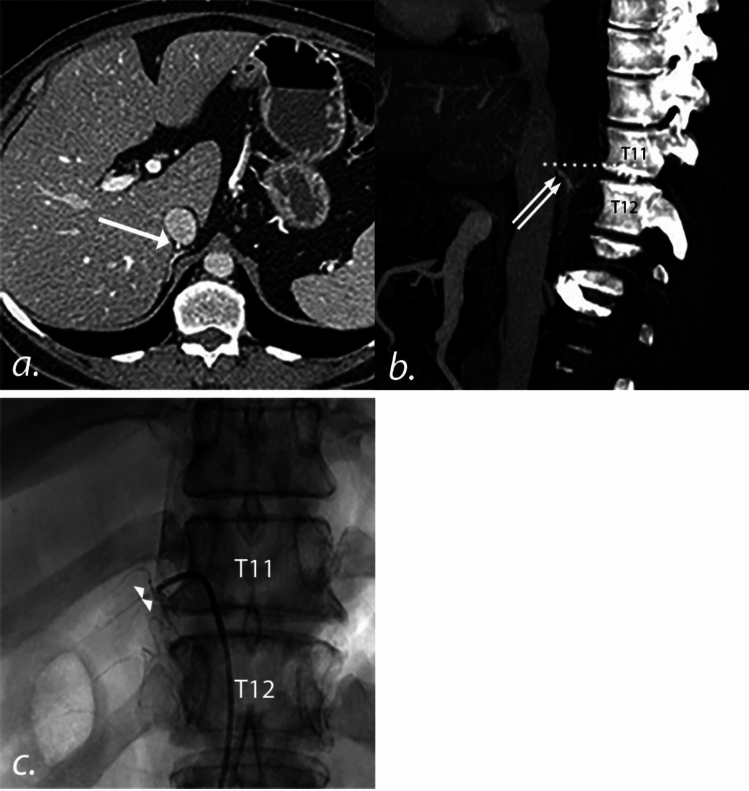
CT imaging and intraprocedural fluoroscopic images during AVS. a Axial CT images from a pre-AVS CT scan demonstrates the origin of the RAV (*arrow*). b Sagittal reformatted CT image from the pre-AVS CT scan demonstrates that the RAV origin (*double arrow*) maps to the lower third of the T11 vertebral body (dotted line). c Intraprocedural fluoroscopic image during AVS demonstrates the RAV (as confirmed with a SI > 3, *double arrowhead*) at the level of the lower third of the T11 vertebral body. *AVS* Adrenal vein sampling, *RAV* right adrenal vein, *SI* selectivity index
